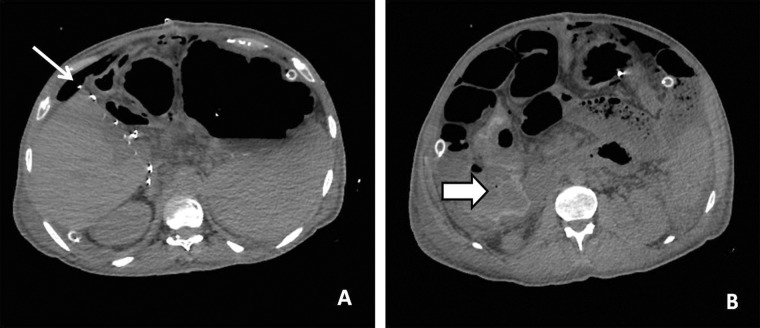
Intestinal perforation. (A,B) Axial non-contrast CT scan images showing free extraluminal air in the peritoneal cavity (arrows) and active contrast extravasation in a case of intestinal perforation (arrowhead).
